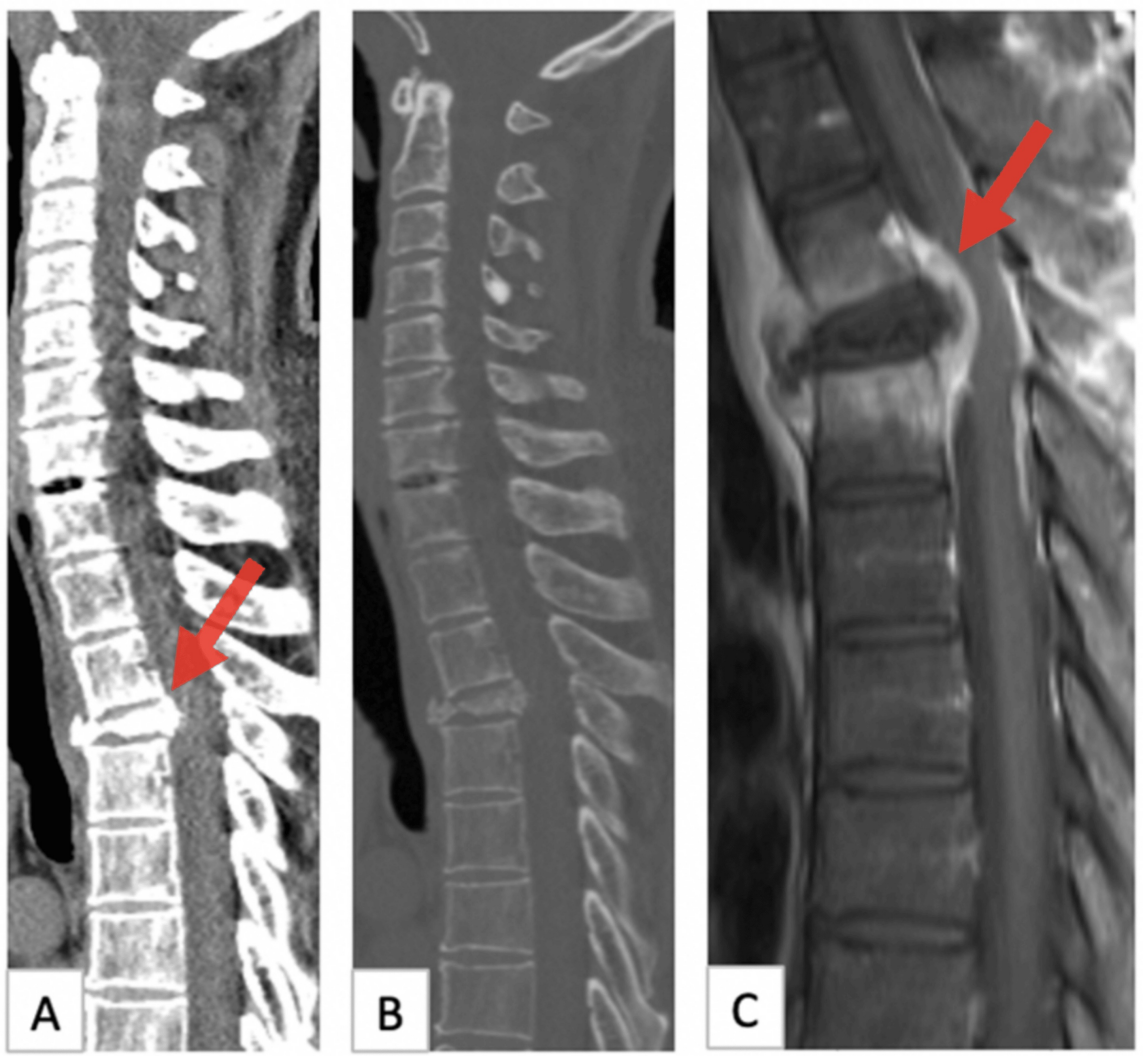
Preoperative images of the patient A, B: Sagittal CT showed compression fracture of four thoracic vertebrae and compression of the spinal canal, C: MR sequence after sagittal contrast showed contrast enhancement of the mass and compression of the spinal canal. T4 vertebral body compression fracture, spinal cord compression (arrow).
